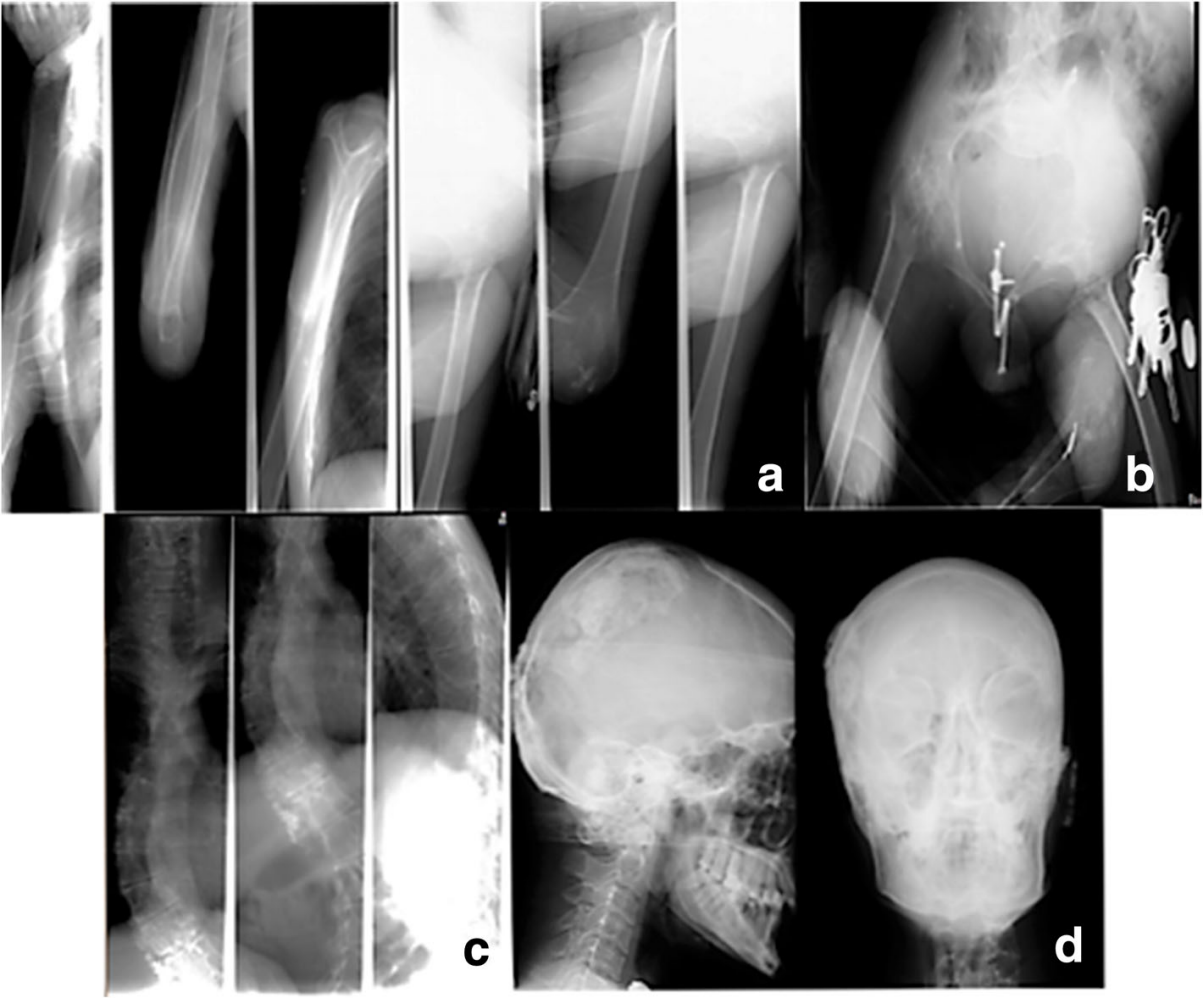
X-rays of patient VI-10 showing (a) radial bone bowing and thin diaphyses, (b) deformity of the iliac bones, thinned pubic rami, severe narrowing of the hip joints, acetabular protrusion, erosion of joint spaces, coxo-femoral ankylosis, thinned fibula, amyotrophy and cutaneous calcifications, (c) thoraco-lumbar scoliosis with paravertebral calcifications at T10, T11 and T12 levels and (d) subcutaneous soft tissue calcifications in the pinna of both ears and in the parietal region of the scalp
